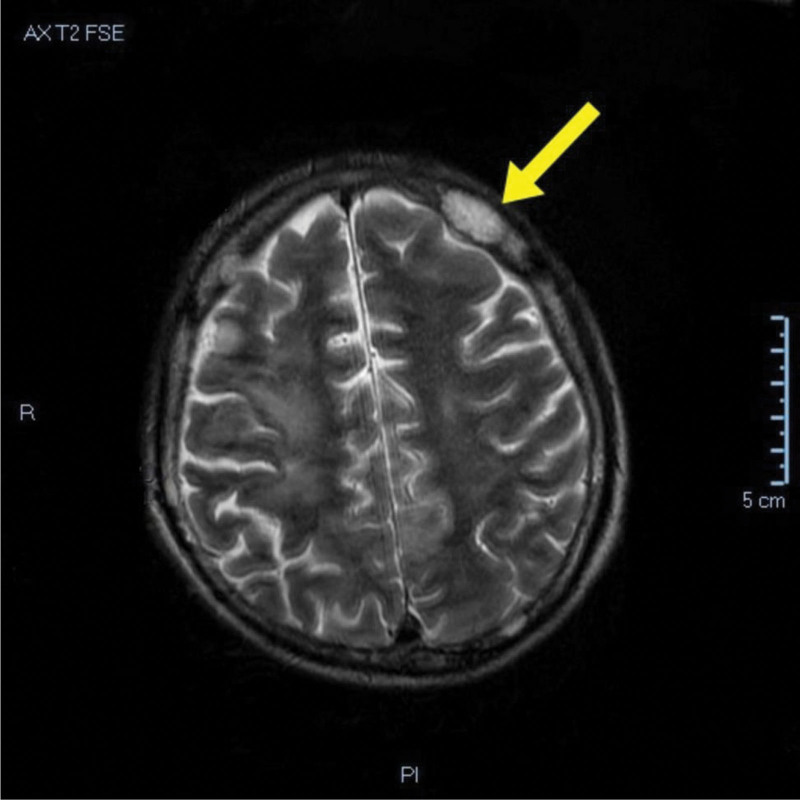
MRI aspect of bone metastasis: nodular lesion with hyperintensity in T2-weighted image. MRI = magnetic resonance imaging.
